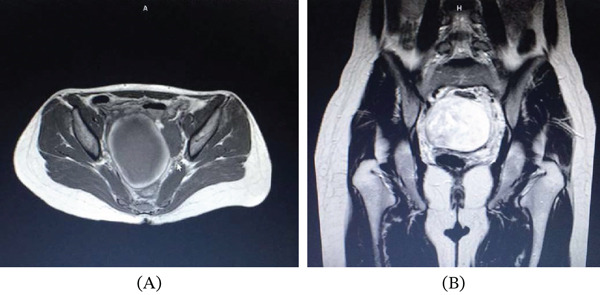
(A) Transverse and (B) coronal view: contrast MRI of the pelvis showing 10‐cm adnexal mass suspicious of right cystadenoma of the ovary with no lymphadenopathies or other notable pelvic lesions.
